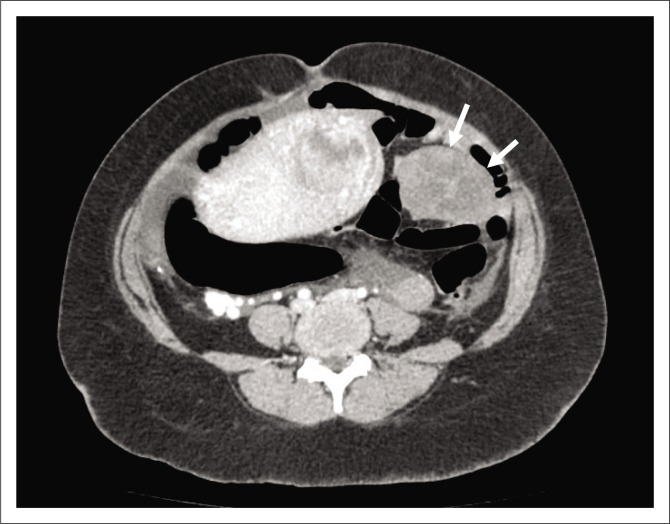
Poorly enhancing enlarged left ovary on axial portal venous computed tomography (arrows).
